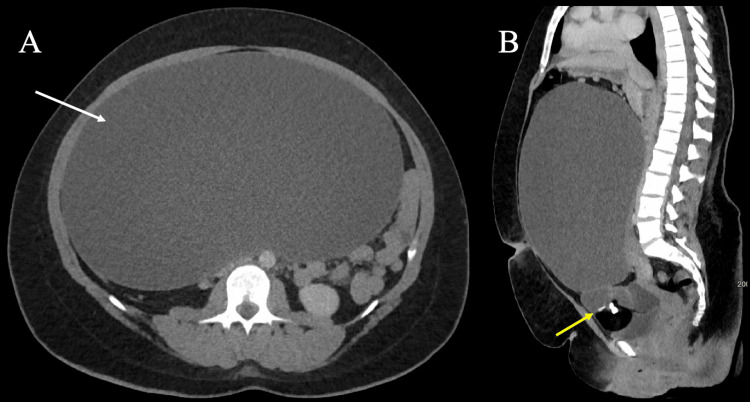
(A) Axial and sagittal CT images of the abdomen/pelvis revealed a 30 x 17 x 30 cm cystic mass (white arrow) spanning the abdomen and displacing the adjacent viscera. (B) The mass appeared to have loculated areas of calcification and fat at the inferior pole (yellow arrow) and large swirling vessels.
